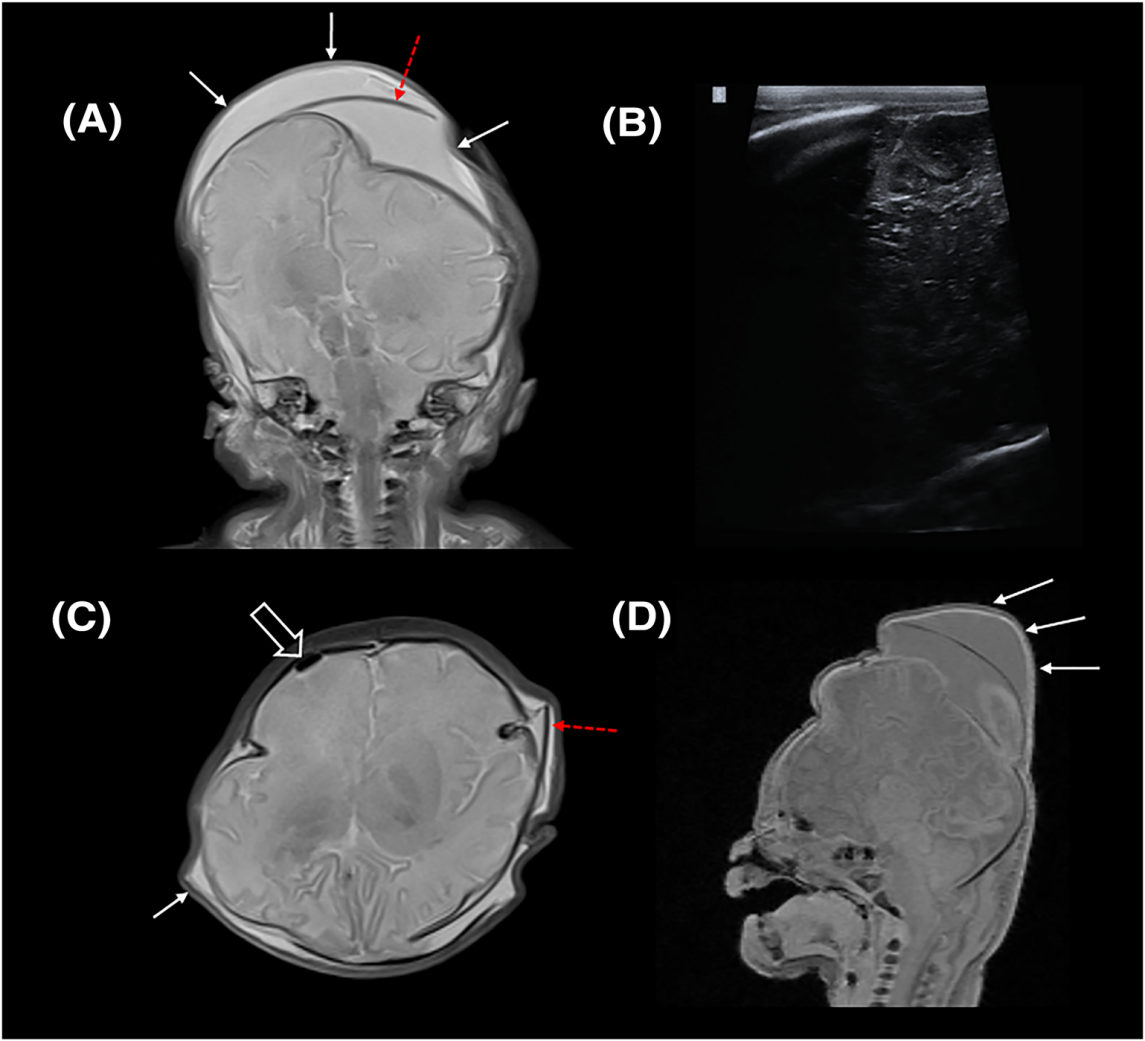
Nondiagnostic post‐mortem ultrasound brain imaging in a 38‐week gestational aged fetus. A, Coronal T2‐weighted post‐mortem MRI image is compared with B, coronal ultrasound image, demonstrating poor sonographic penetrance and visualisation of the right cerebral hemisphere. The MRI image demonstrates marked soft tissue scalp oedema (white arrows) and cranial sutures overlapping (red dashed arrow) contributing to the poor ultrasound quality. Further, C, axial T2‐weighted and D, sagittal T1‐weighted MRI images reveal a locule of intracranial gas (open arrow) and further areas of oedema (white arrow) and sutural disruption (red dashed arrow) [Colour figure can be viewed at http://wileyonlinelibrary.com]
